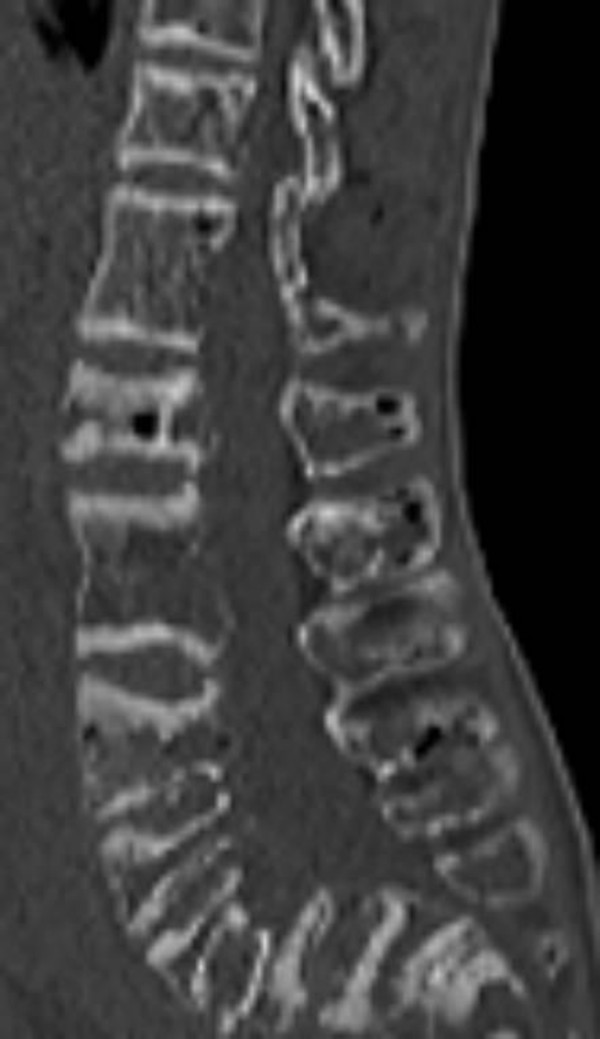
Sagittal 3DCT scan of the thoracolumbar radiograph showed a combination of deformities ranged from severe flattening, fusion, shrinkage and compression fractures. A feature mimicking anisospondyly in skeletally dysplastic patients (Different abnormal shapes of the vertebral bodies) was evident.
